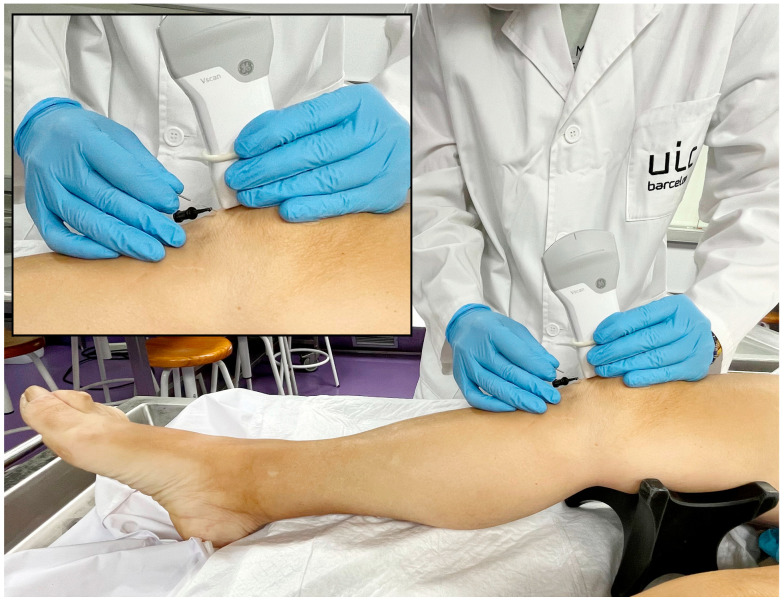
Performance of the ultrasound-guided percutaneous electrolysis technique and subject positioning.
